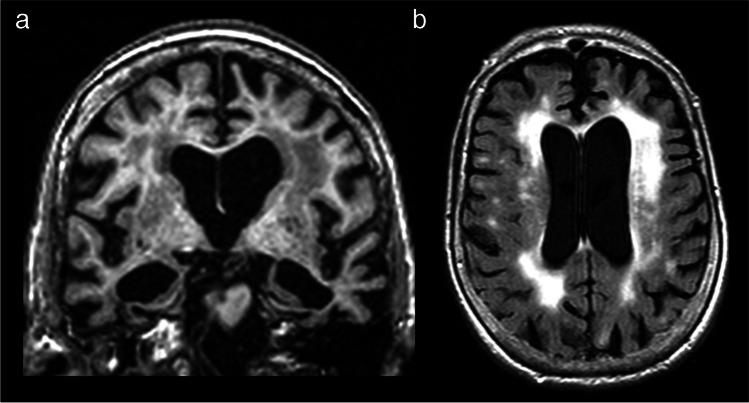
Representative case of a 91 year-old male patient with AD. (a) Coronal reformat of a 3D T1-weighted sequence shows severe widening of the choroid fissures and severe enlargement of the temporal horns, with marked atrophy of both hippocampi (medial temporal lobe atrophy – MTA – score of 4). (b) Axial T2-FLAIR sequence reveals hyperintensities involving the periventricular and the deep white matter, with irregular periventricular signal extending to the deep white matter in the former and severe confluence in the latter (periventricular and deep Fazekas score of 3)
